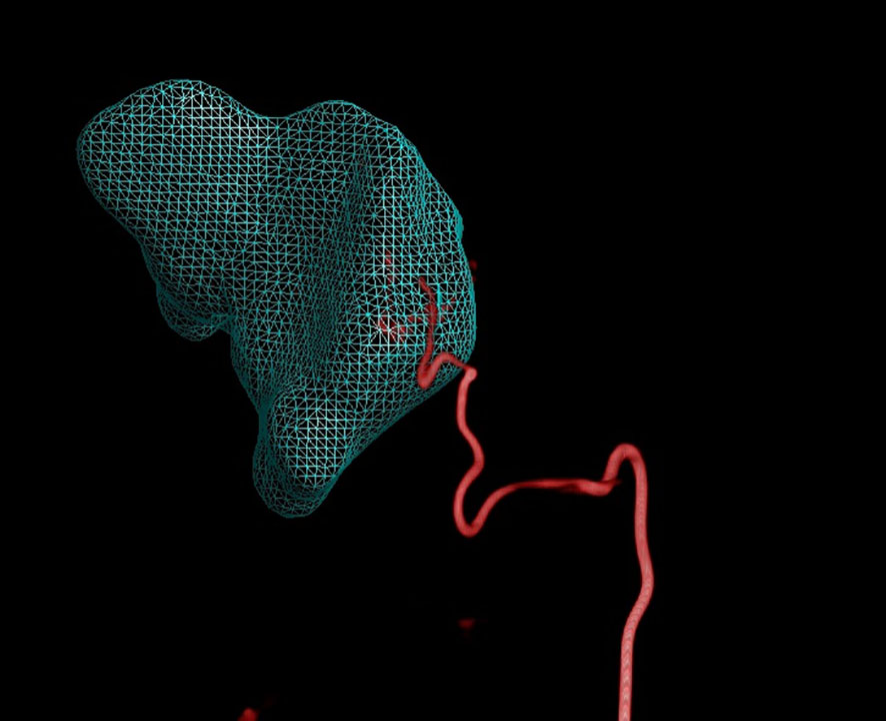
Dual CBCT used to create a 3D reconstruction model of its blood supply vessels (viewed from a left anterior oblique position) after successful super-selection.
